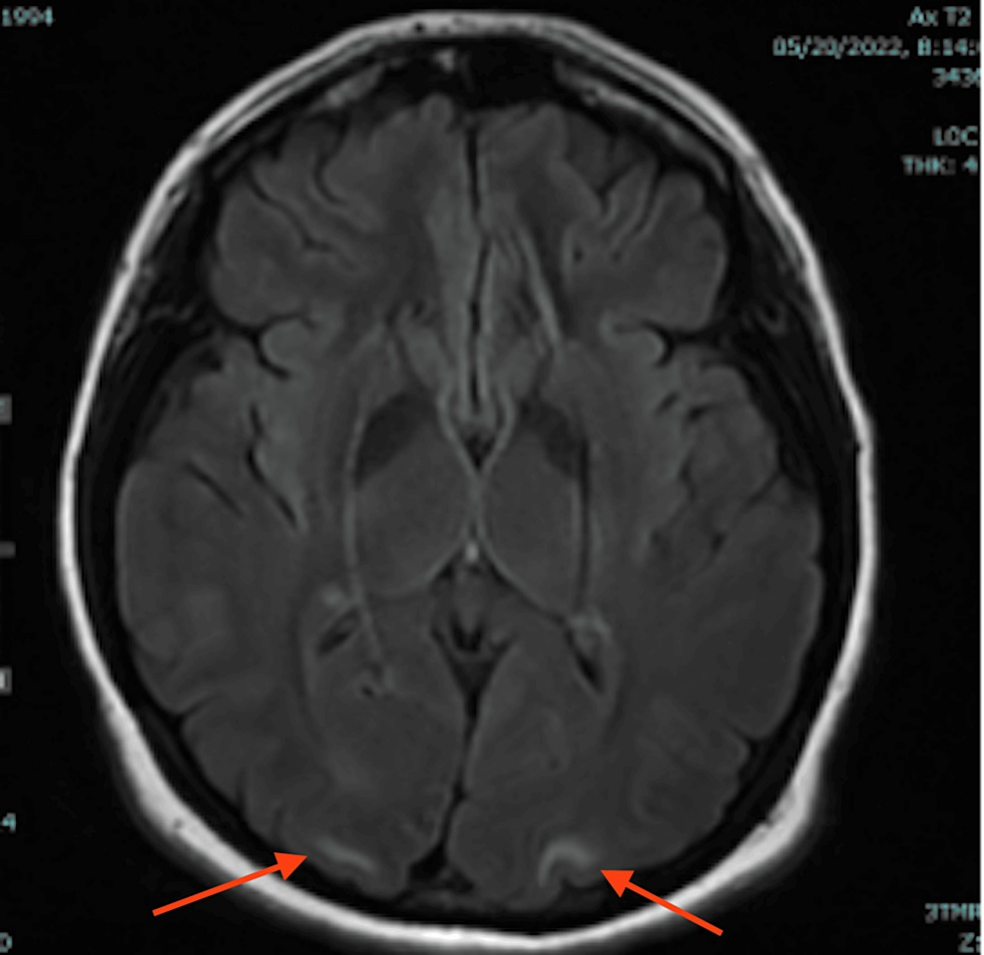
T2/FLAIR MRI of the brain (image II) FLAIR: Fluid attenuated inversion recovery Red arrows show hyperintensities in bilateral occipital lobes.
